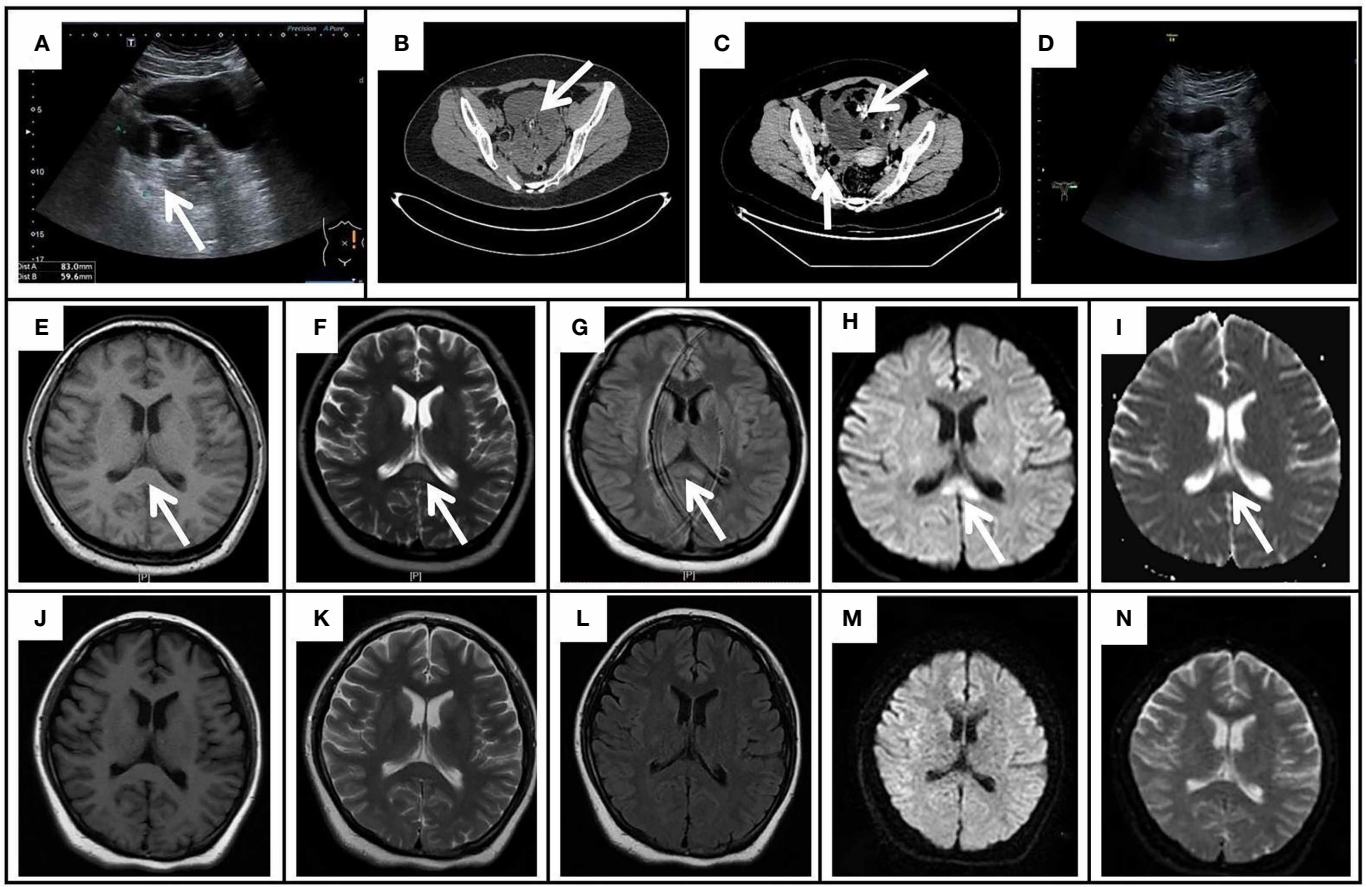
The gynecological ultrasound, pelvic CT, and cranial MRI results of the patient. (A) The gynecological ultrasound showed a mixed echogenic mass (8.3 cm × 5.9 cm) in the left ovary. (B) Pelvic CT showed an irregular cystic mass (10.2 cm × 8.4 cm) with a few calcifications and fatty components in the posterior aspect of the bladder. (C) Enhanced CT of the pelvis revealed a large cystic-solid mass in the anterior aspect of the uterus with no enhancement of the cystic component, fat and calcification, and mild enhancement of the solid component; A mass of uneven density on the right side of the uterus with a predominantly solid component, mild enhancement of the solid component and no enhancement of the fatty component. (D) The repeated gynecological ultrasound demonstrated no abnormal echogenicity. Initial cranial MRI exhibited an isolated splenium of the corpus callosum (SCC) lesion (arrows) with slight hypointensity on T1WI (E), hyperintensity on T2WI (F), FLAIR (G), and DWI (H), and hypointensity on the ADC map (I). On day 52 after onset, repeated MRI demonstrated the lesion of SCC lesion was completely resolved on T1WI (J), T2WI (K), FLAIR (L), DWI (M), and ADC (N).
